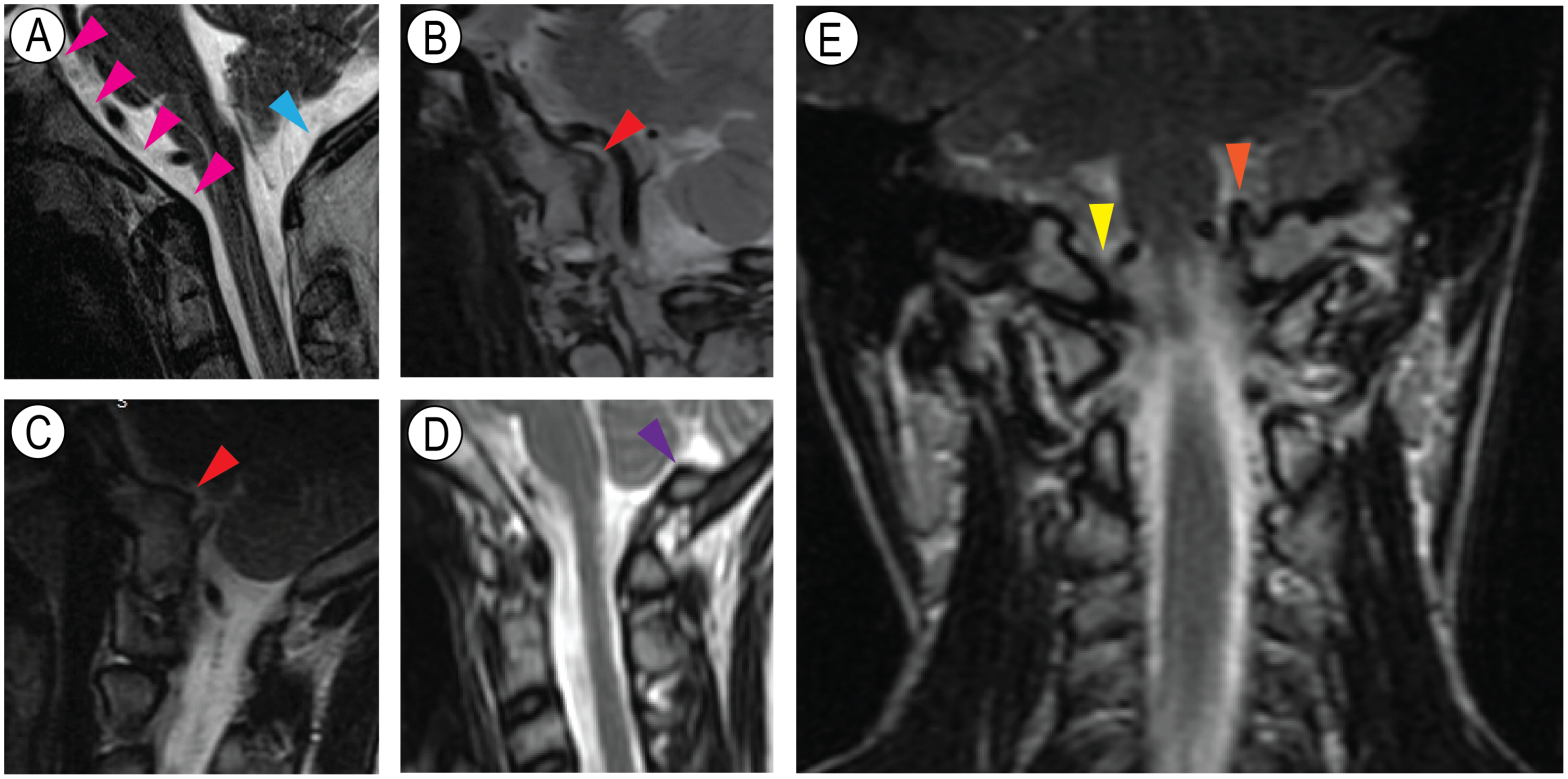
HME patients have cranial base defects. (A) Sagittal plane T2 MRI sequence showing an example of a normal clivus (delineated by pink arrowheads) and normal posterior foramen magnum (cyan arrowhead) in a HME patient. (B-C) Sagittal plane T1 and T2 MRI sequences showing a very large osteochondroma-like outgrowth (red arrowheads) in a patient that is characterized by continuity with clivus cortical bone and marrow. For orientation, note that the cerebellum is the large tissue mass occupying the upper right half of the images. (D) Sagittal T2 MRI sequence from a patient displaying an irregularity in the occipital lip of the foramen magnum (purple arrowhead). (E) Coronal plane T2 MRI sequence showing an osteochondroma-like outgrowth (orange arrowhead) and a more normal contralateral side (yellow arrowhead).
